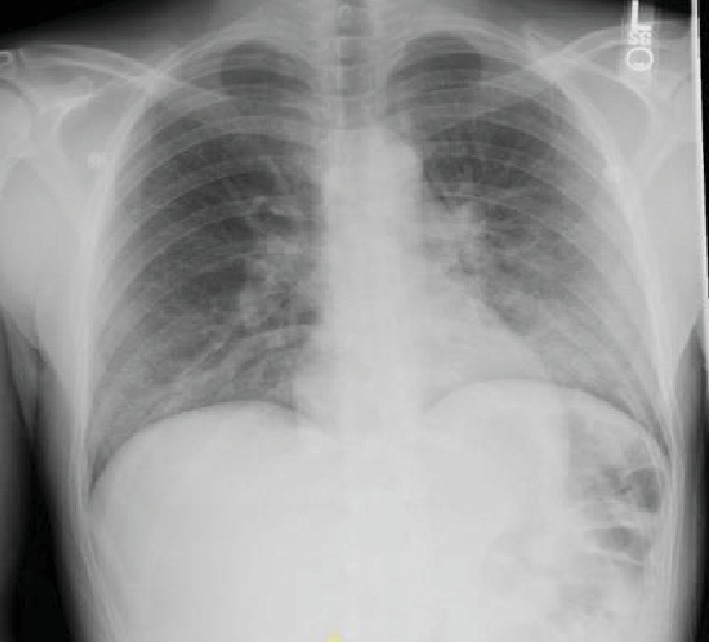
Chest X-ray showing bilateral airspace opacities.
